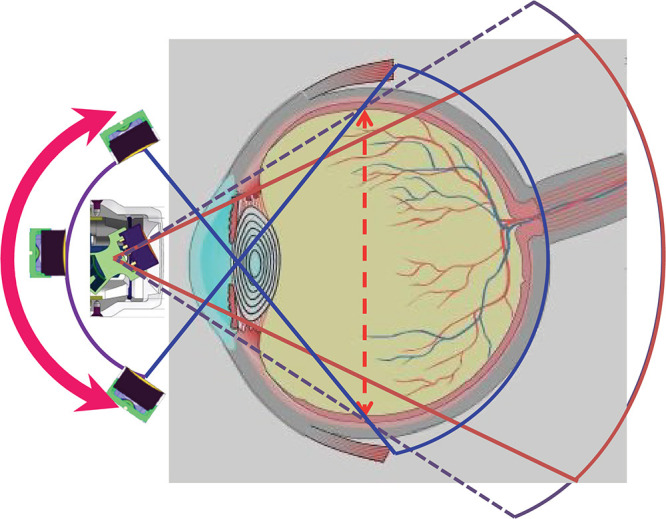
Arc-scan image obtained using a wide-angle ultrasound diagnostic device The wide-angle ultrasound diagnostic device can obtain images over a wider range, indicated by blue lines, compared with the conventional ultrasound diagnostic B-mode device, indicated by red lines
